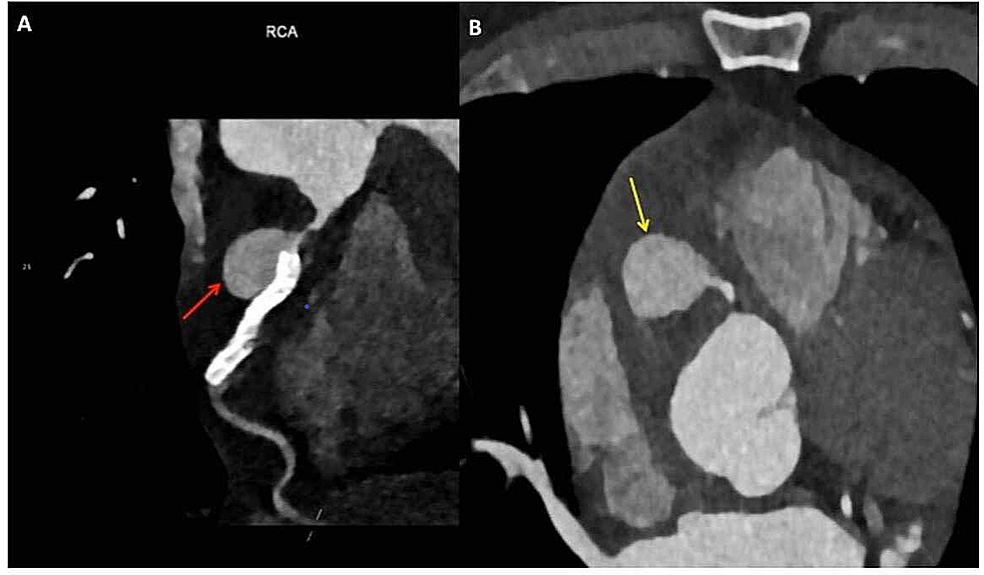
CT coronary angiography (A) CT coronary angiography oblique view of the right coronary artery (RCA) shows the large pseudoaneurysm at the proximal end of the stent arising approximately 12 mm from the ostium (red arrow); (B) CT coronary angiography axial view at the level of aortic sinus shows the pseudoaneurysm along the course of the RCA (yellow arrow).
